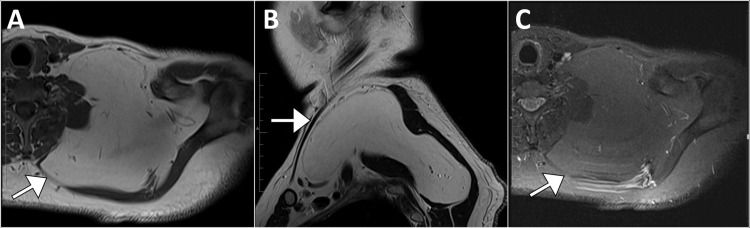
Magnetic resonance imaging (Panel A shows an axial T1-weighted image without fat saturation, Panel B shows a sagittal T1-weighted image without fat saturation, and Panel C shows an axial T1-weighted image with fat saturation) showing a large bilobed intramuscular mass within the left trapezius muscle, following fat signal intensity on all sequences with complete suppression on short tau inversion recovery images. The lesion measures 13.5 cm in anterior-posterior diameter, 16.5 cm transversely, and 13.1 cm longitudinally. Despite its size, the lesion shows no significant post-contrast enhancement or concerning features. It extends into the subacromial fat and around the supraspinatus muscle but does not infiltrate the rotator cuff. The lesion abuts the posterior aspect of the acromioclavicular joint without intra-articular involvement, and is inseparable from the cervical neurovascular bundle, with vessels from the subclavian artery traversing the mass.
